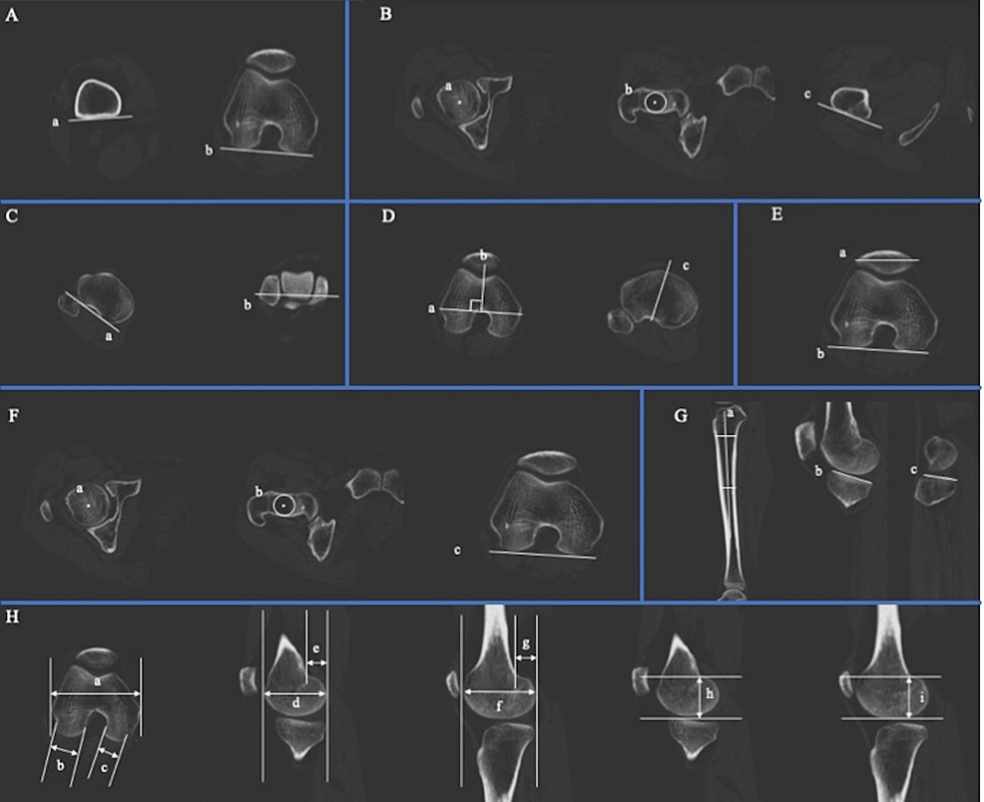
CT measurement parameters. Measured with ZedKnee® system (LEXI, Tokyo, Japan). (A) Distal femoral torsion. a: posterior tangent line of the femoral shaft; b: posterior femoral condyle tangent line. (B) Proximal femoral torsion. a: center of the femoral head; b: center of the circle tangent to the femoral neck; c: lesser trochanter tangent. (C) Tibial torsion. a: posterior tibial tangent; b: line connecting the medial and lateral malleolus of ankle. (D) Femoral tibial joint torsion. a: Surgical Epicondylar Axis (SEA); b: vertical line of the SEA; c: line connecting the PCL attachment to the medial edge of the tibial tuberosity. (E) Patellar tilt. a: patellar axis; b: posterior femoral condyle tangent line. (F) Femoral anteversion angle. a: center of the femoral head; b: center of the circle tangent to the femoral neck; c: posterior femoral condyle tangent line. (G) Medial and lateral posterior tibial slope angles. a: tibial sagittal axis; b: medial tibial plateau tangent; c: lateral tibial plateau tangent. (H) Femoral condyle measurements. a: medial-lateral width of the epicondyle; b: lateral femoral posterior condyle width; c: medial femoral posterior condyle width; d: medial femoral condyle anteroposterior diameter; e: medial femoral posterior condyle anteroposterior diameter; f: lateral femoral condyle anteroposterior diameter; g: lateral femoral posterior condyle anteroposterior diameter; h: medial femoral condyle height; i: lateral femoral condyle height.
